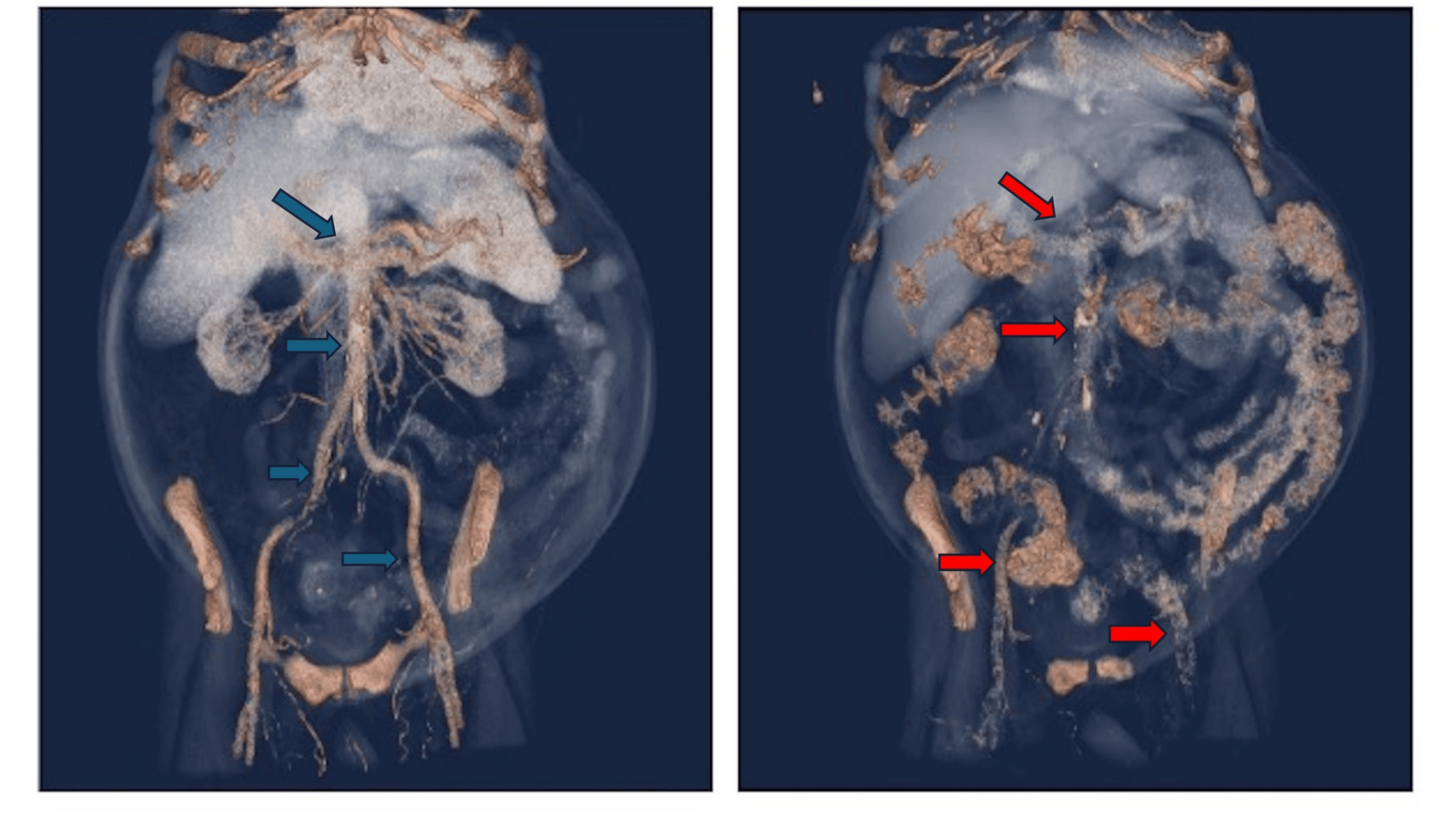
Coronal maximal intensity projection reformatted computed tomography (CT) and anterior projection 3-dimensional volume rendering. Images were obtained with a time difference of 3 years. On baseline examination (right image), there was moderate diffuse calcified atherosclerosis affecting the abdominal aorta and main abdominal and pelvic branches (red arrows). Follow-up examination (left image) showed significant interval worsening especially involving splanchnic and mesenteric vasculature, bilateral iliac axis and deep pelvic branches (blue arrows).
